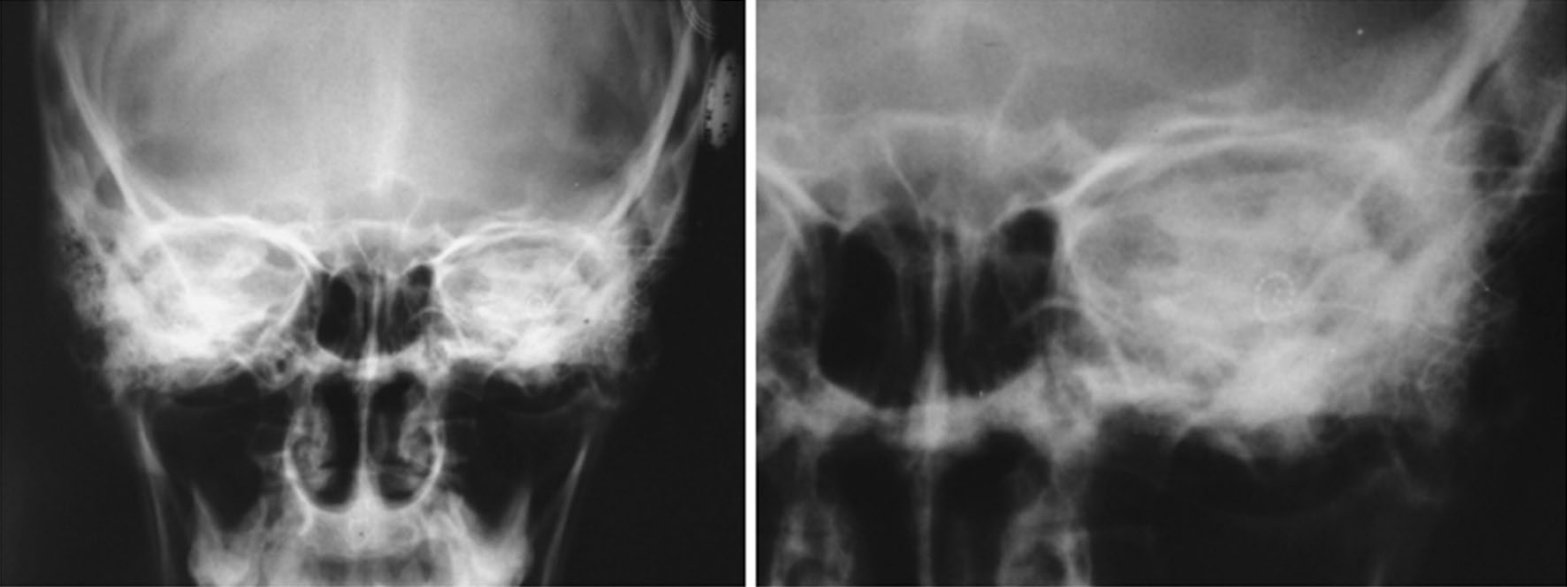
Post-operative transorbital X-Ray. Observe the positioned electrode inside the left cochlea.
